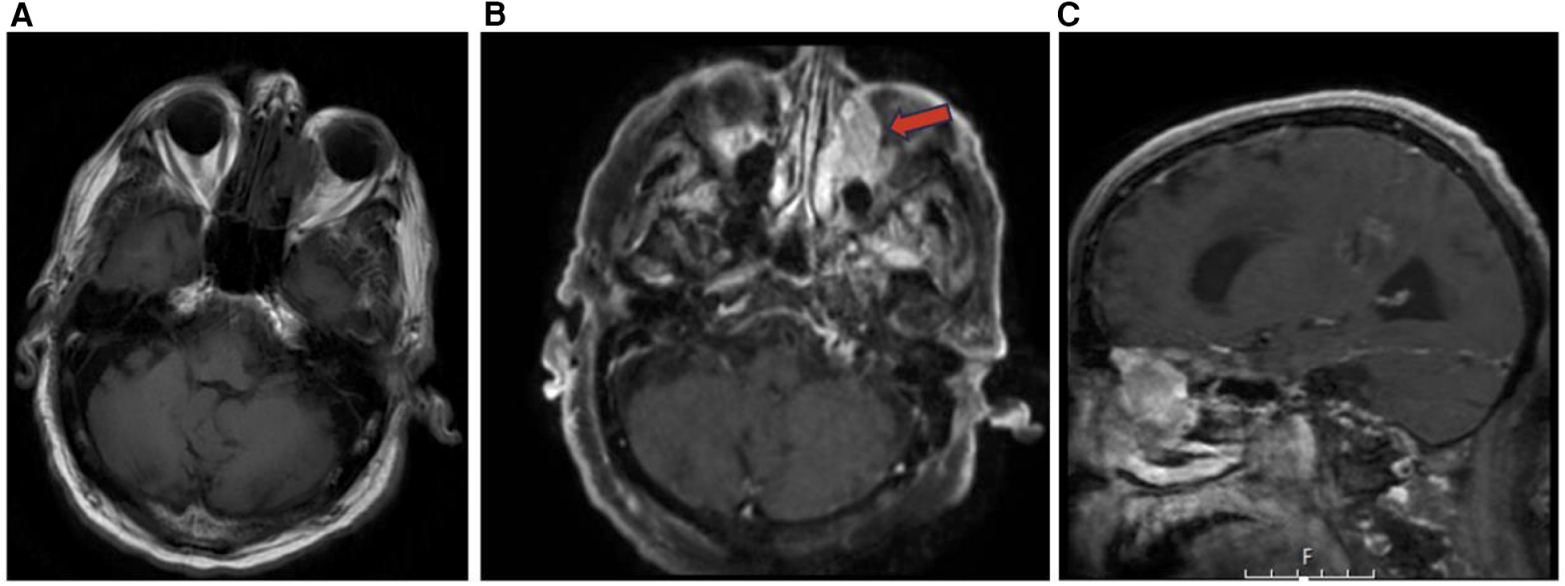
Adenocarcinoma MRI axial T1 (A) axial T1 + C (B) and sagittal T1 + C (C) of an invasive sinonasal carcinoma in the left ethmoid to extraconal orbital region (red arrow) likely representing a primary sinonasal adenocarcinoma.
